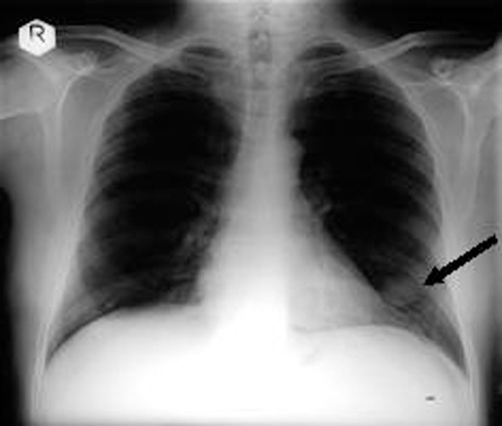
Chest radiograph showing a ‘coin shaped lesion’ in the lower lobe of left lung.
